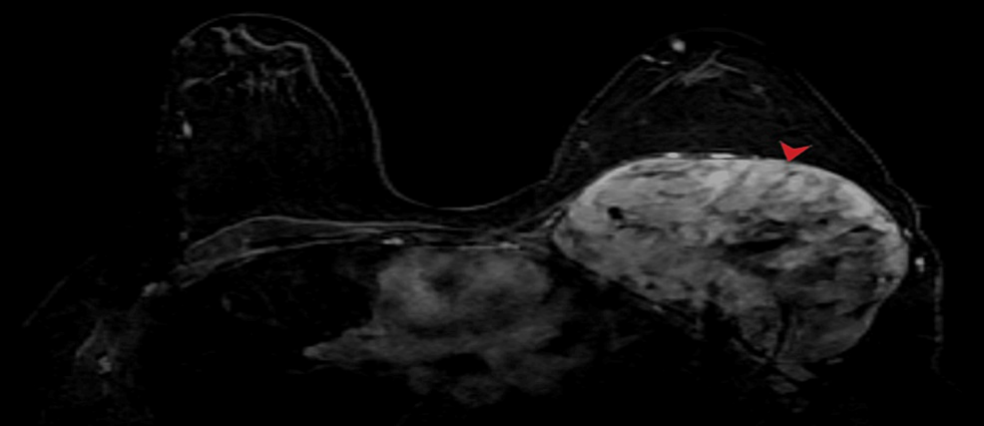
Post-contrast fat suppressed T1 axial MR image shows a heterogeneous post-contrast enhancement pattern due to presence of intra-tumoral necrotic changes that remain unenhanced.
